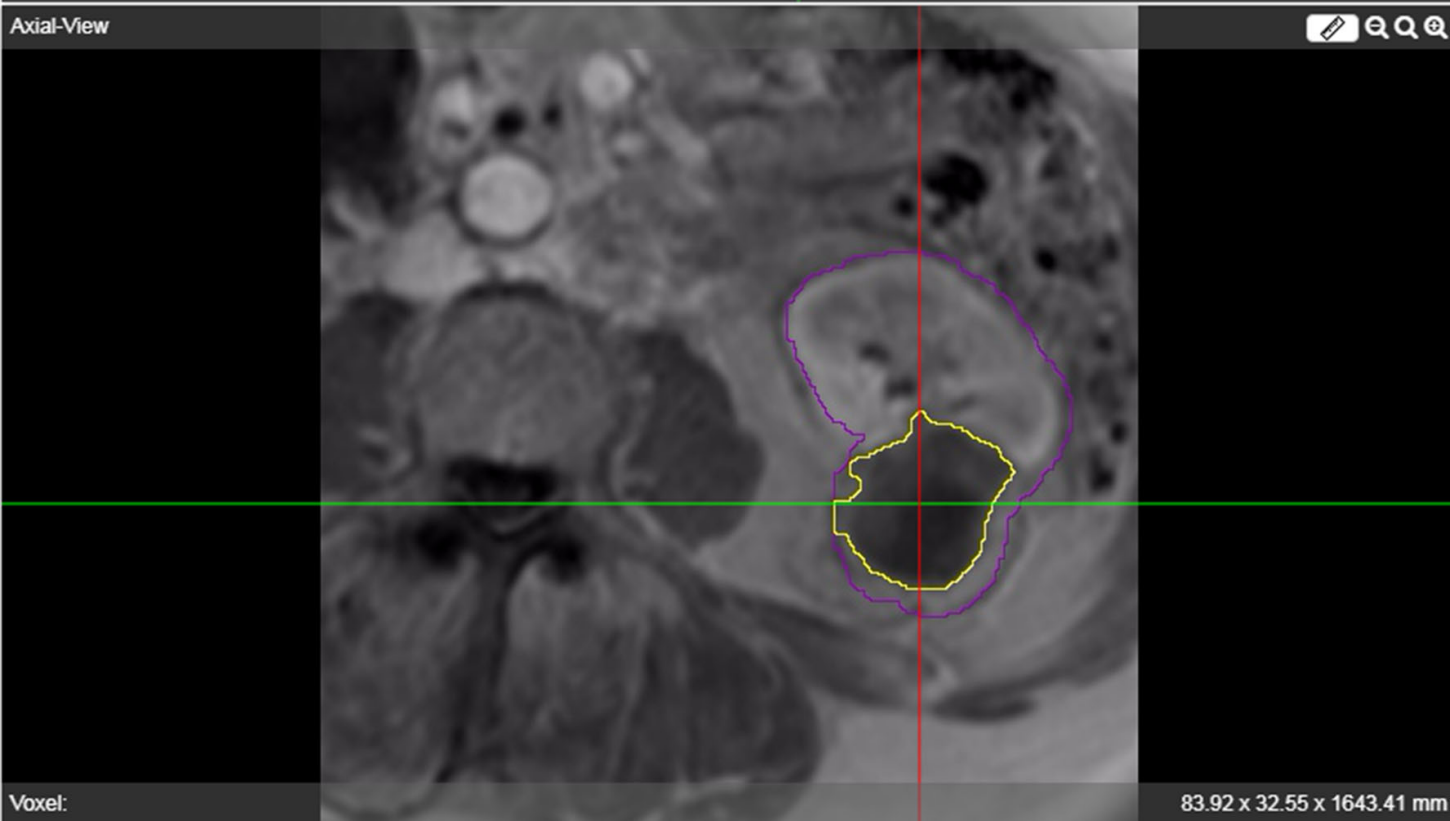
Example of segmented kidney (purple) and ablated ablation zone (yellow) on 1-month follow-up MRI scan
